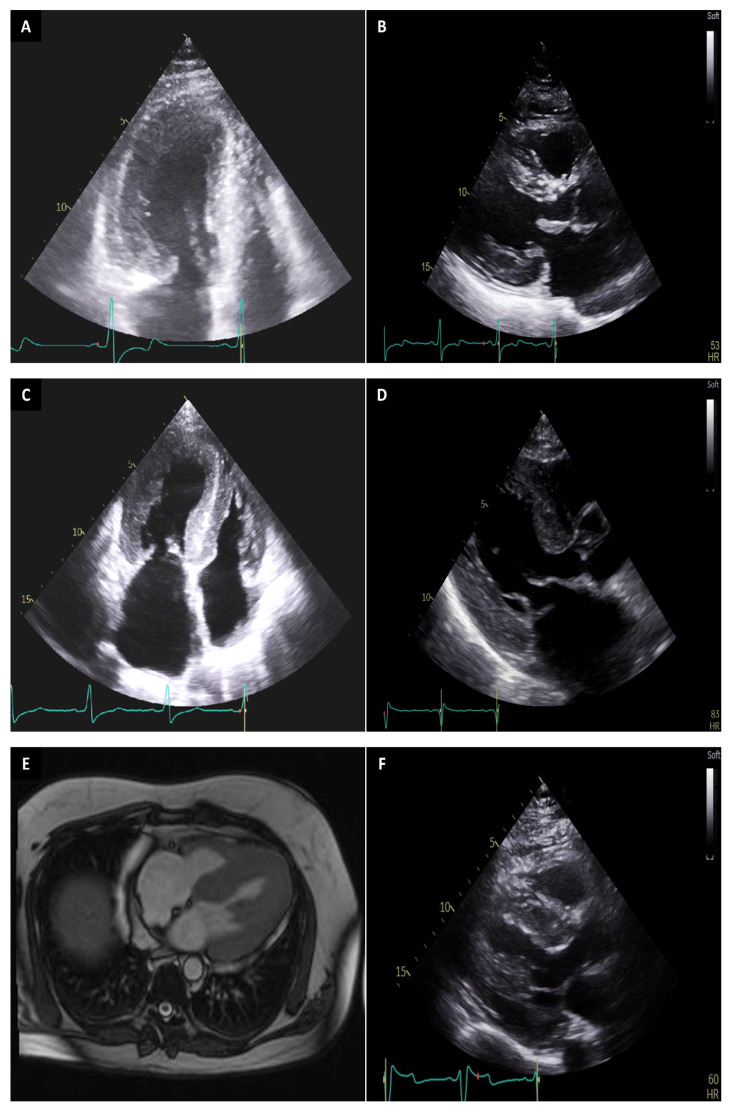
Cardiovascular imaging revealing hypertrophic cardiomyopathy (HCM) and phenocopies. Panel A and B show apical 4-chamber (A) and parasternal long axis (B) transthoracic echocardiography (TTE) views demonstrating asymmetric septal hypertrophy (reverse curve morphology, maximal wall thickness 19 mm) in a patient with confirmed typical HCM. Panel C and D depict apical 4-chamber (C) and parasternal long axis (D) TTE views showing increased wall thickness of the left and right ventricle and enlarged left atrium in a patient with light chain (AL) amyloidosis. Panel E and F show images from a patient initially diagnosed with HCM; however, genetic testing confirmed the diagnosis of Fabry’s disease in the absence of any other clue for Fabry; magnetic resonance imaging coronal section (E) reveals diffuse left ventricular hypertrophy and parasternal long axis TTE view (F) shows increased wall thickness.
